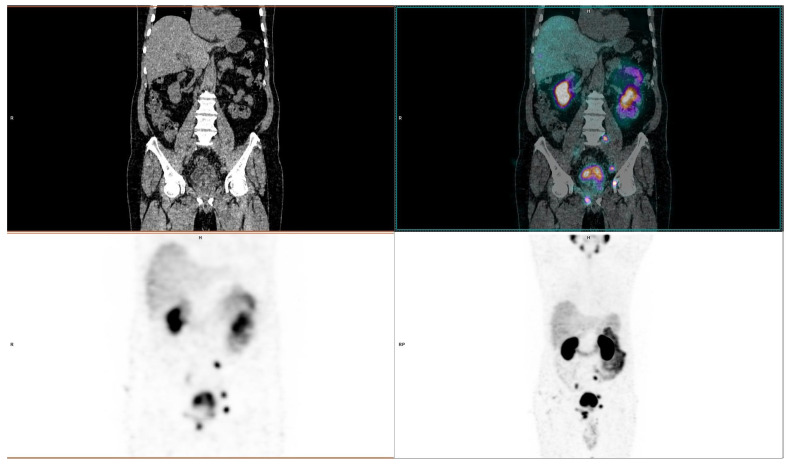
A 61-year-old man with BCR of prostate cancer after radiation therapy (ISUP grade 3) underwent Tc-99m-PSMA SPECT/CT at a PSA of 6.33 ng/mL, which revealed multiple sites pathological tracer uptake in the pelvic and retroperitoneal lymph nodes, as well as pelvic bones, compatible with prostate cancer metastases. ADT was initiated afterwards as a part of routine clinical management, which resulted in a decrease in serum PSA. On follow-up abdominopelvic MDCT after 5 months, reported enlarged lymph nodes on Tc-99m-PSMA SPECT/CT were no longer visible, indicating disease regression; pelvic bones still had previously reported sclerotic lesions, stable in size.
